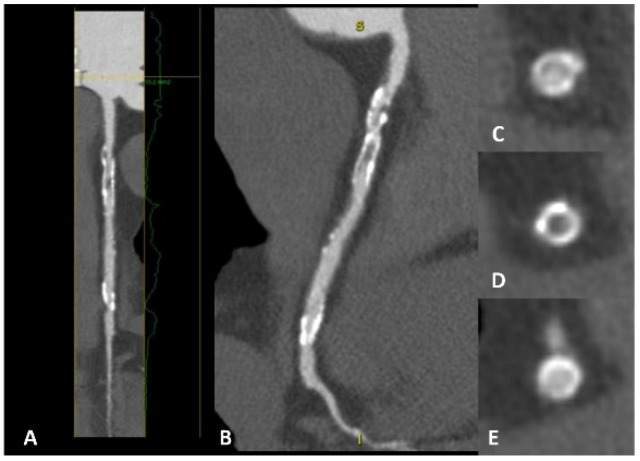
A patient with previous percutaneous revascularization of the right coronary artery, with cardiac CT showing evident in-stent restenosis in both long-axis view (panels A,B) and short-axis view (panels C–E). In panel (D), a clear hypodensity is evident inside the stent lumen compared with panels (C,E).
